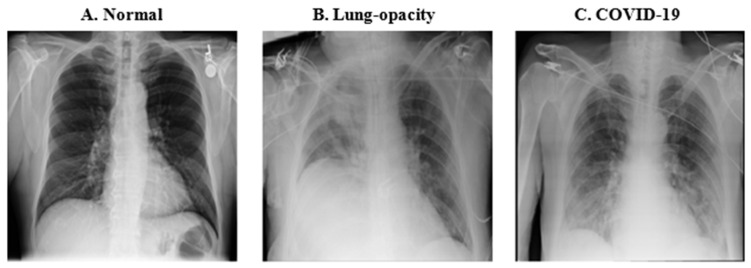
Chest X-ray figures used for the training. (A). (Normal fig.) A normal chest X-ray of a healthy individual. (B). (Lung-opacity fig.) A patient with unilateral right-sided opacity from another cause. (C). (COVID-19 fig.) A COVID-19 patient with bilateral opacities consistent with COVID-19 pneumonia.
